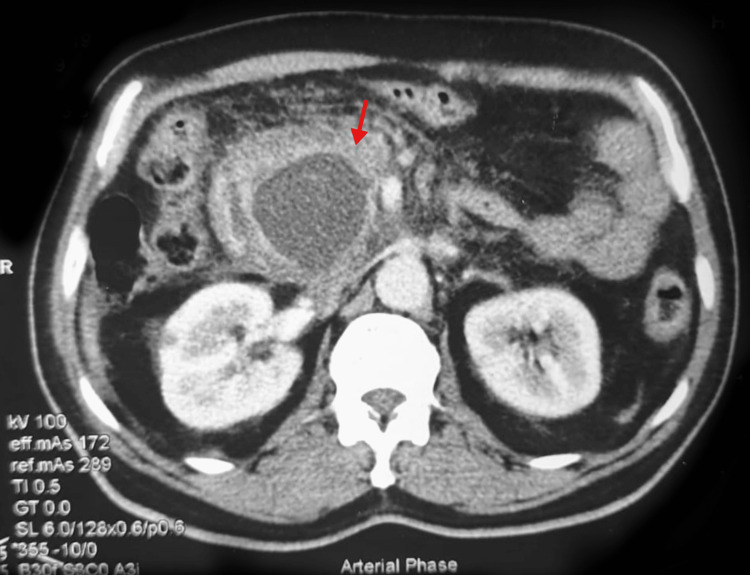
Pseudocyst in the pancreas on CT scan abdomen
